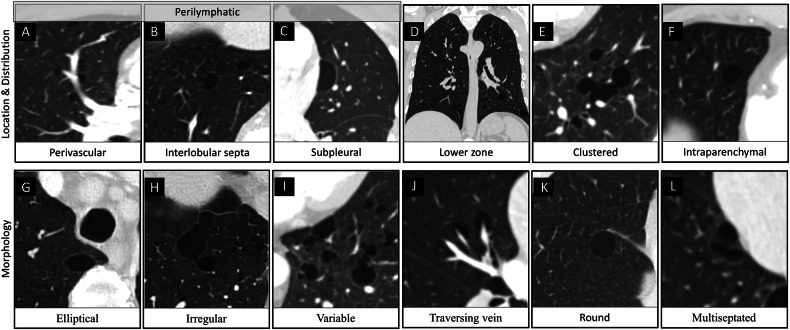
CT characteristics of pulmonary cysts: *perivascular location* (axial plane, A); *interlobular septal* location (axial plane, B); *paramediastinal location* (axial plane, C); *perilymphatic-predominant* distribution (A–C); *lower zone predominant* distribution (coronal reformat, D); *clustered* (axial plane, E); *intraparenchymal* location (axial plane, F); *elliptical-shape* morphology (axial plane, G); *irregular-shaped* morphology (axial plane, H); *variable* morphology (axial plane, I); *traversing vein* sign (axial plane, J); *round-shaped* morphology—a symmetric cyst with a diameter ratio of long to short axes < 1.1 (axial plane, K); *multiseptated cyst* (axial plane, L). Note that all cysts are thin-walled
